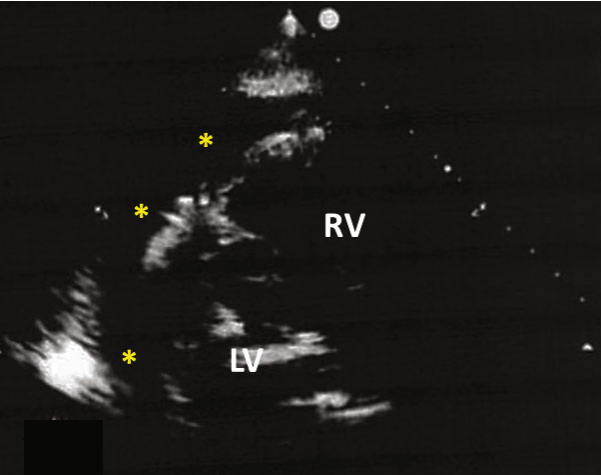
Echocardiography findings of a substantial pericardial effusion (yellow asterisks). LV = left ventricle, RV = right ventricle.
